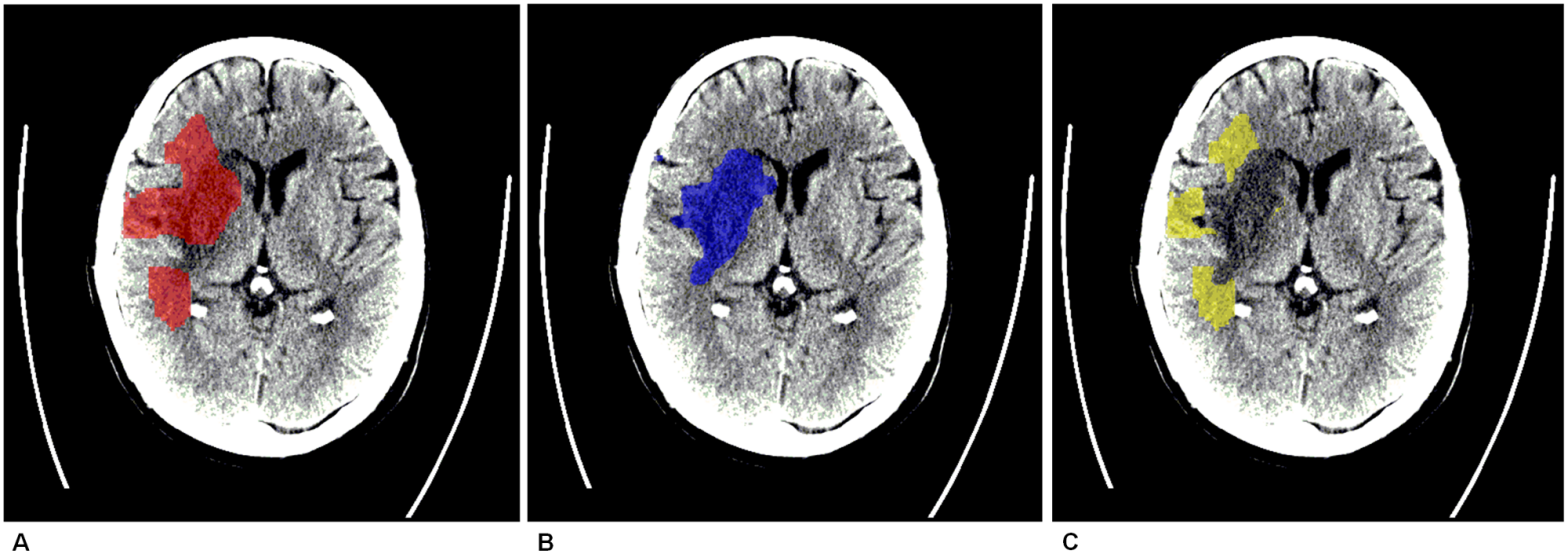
Example of a misclassified ischemic core. A. Ischemic core as selected by CT perfusion software on baseline CTP (projected on follow-up noncontrast CT). B. Final infarct as determined on follow-up noncontrast CT. C. Misclassified ischemic core region projected on follow-up noncontrast CT.
